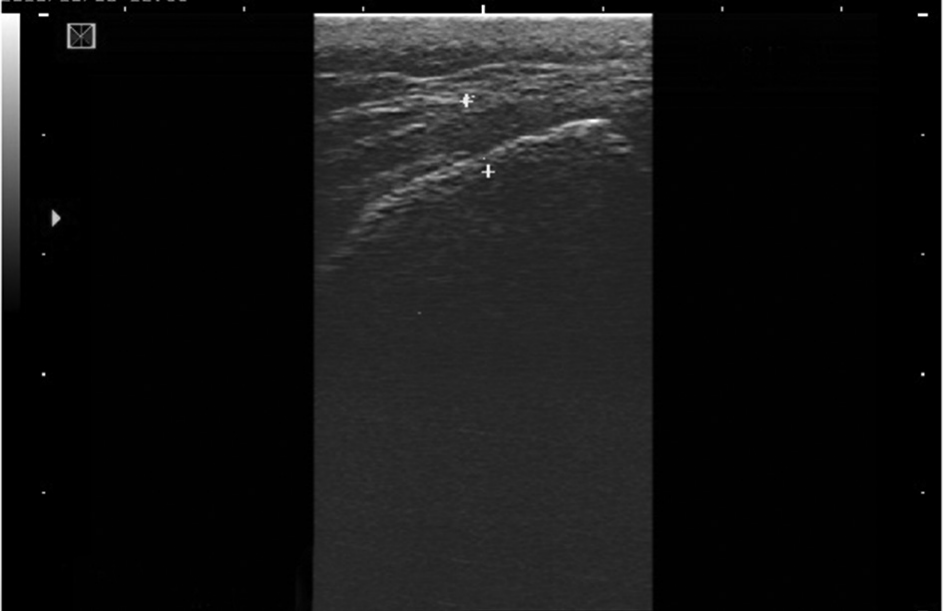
Longitudinal view of the sacral hiatus by ultrasound, two points refer to the diameter of the sacral canal at the apex.
